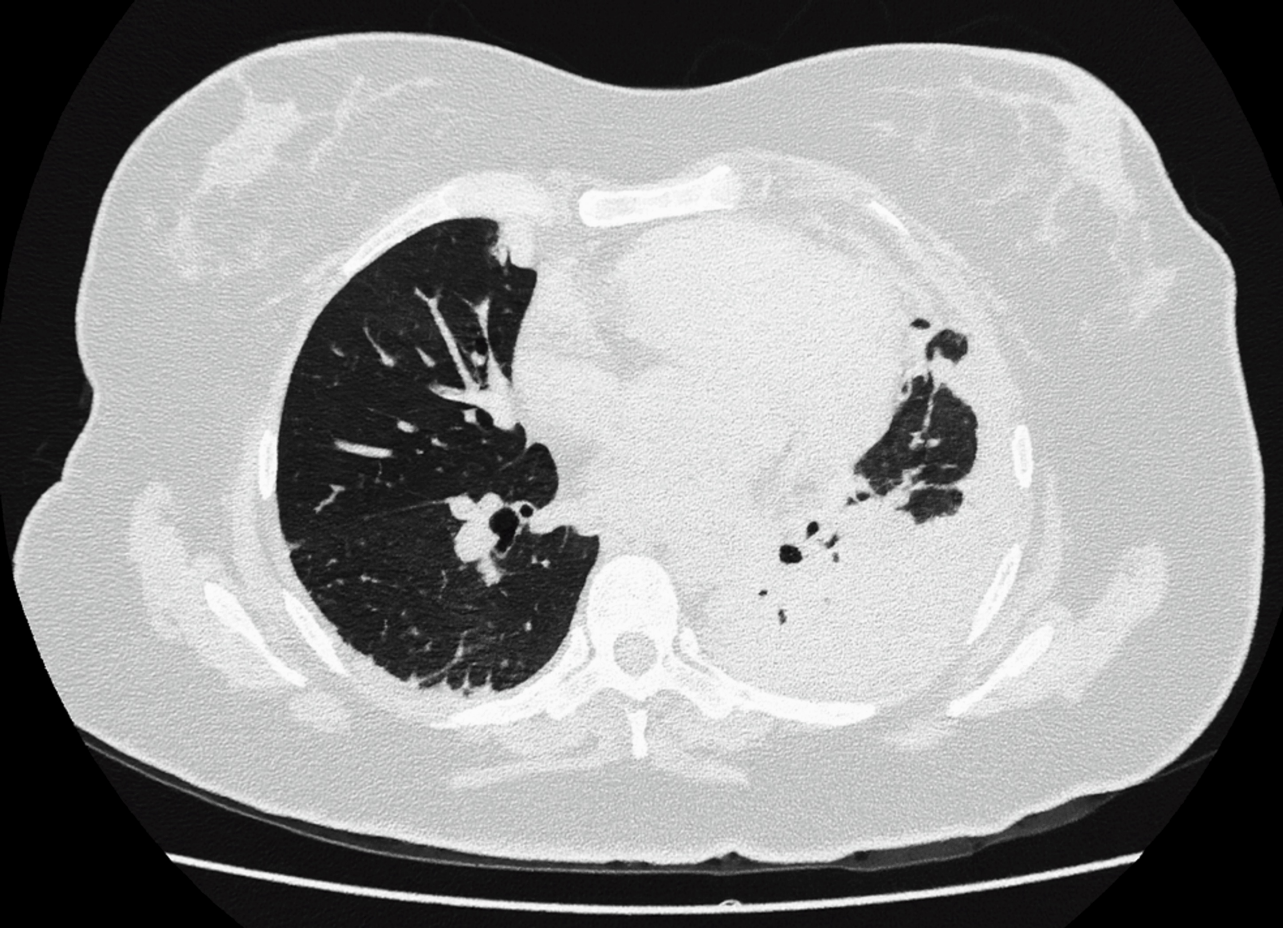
Non-contrast chest computed tomography (CT) scan Non-contrast chest computed tomography (CT) scan showing a left pleural effusion with loculated features and basal consolidation in the left lower lobe, along with ill-defined areas of consolidation in the right lower lobe.
